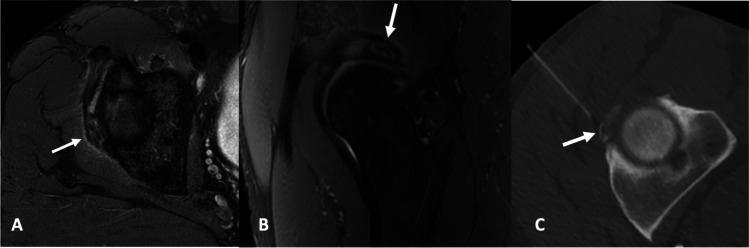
A 47-year-old male presented with an acute onset of severe anterior hip pain. A, B Axial and sagittal proton density sequences with fat saturation demonstrate superolateral intra-labral calcification (arrow) and adjacent mild paralabral edema. C Axial CT image obtained during an image-guided corticosteroid injection best demonstrates the intra-labral calcification (arrow)
